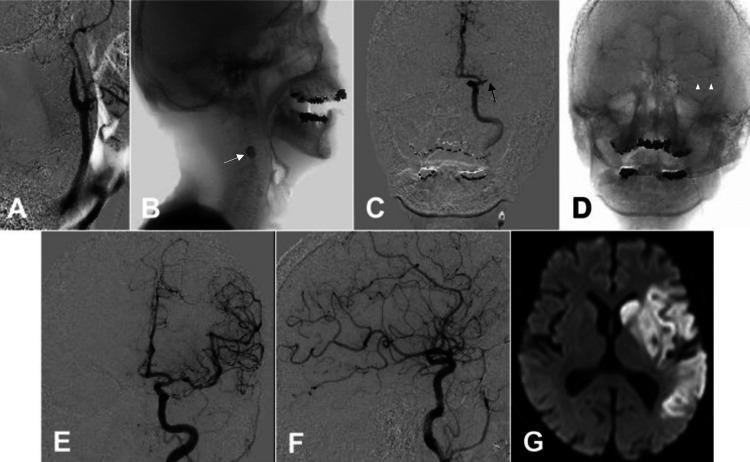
Intraprocedural cervical and cranial angiogram and postprocedural magnetic resonance imaging (MRI). A. A lateral view of the left common carotid angiogram showing occlusion of the origin of the left ICA. B. Inflation of the balloon of the Optimo 8 Fr (Tokai Medical Products, Aichi, Japan) (white arrow) was followed by a decrease in systolic blood pressure. C. An anteroposterior view of the left internal carotid angiogram showing occlusion in the left MCA (black arrow). D. An Embotrap III 5 × 37 mm (Cerenovus, Fremont, CA, USA) (white arrowheads) was used. E, F. Angiograms showing complete recanalization of the left ICA and intracranial arteries, with TICI score of 3; E. Cranial anteroposterior view, F. Cranial lateral view. G. Diffusion-weighted MRI showing cerebral infarction in the area of the left ICA. ICA, internal carotid artery; MCA, middle cerebral artery; TICI, thrombolysis in cerebral infarction
